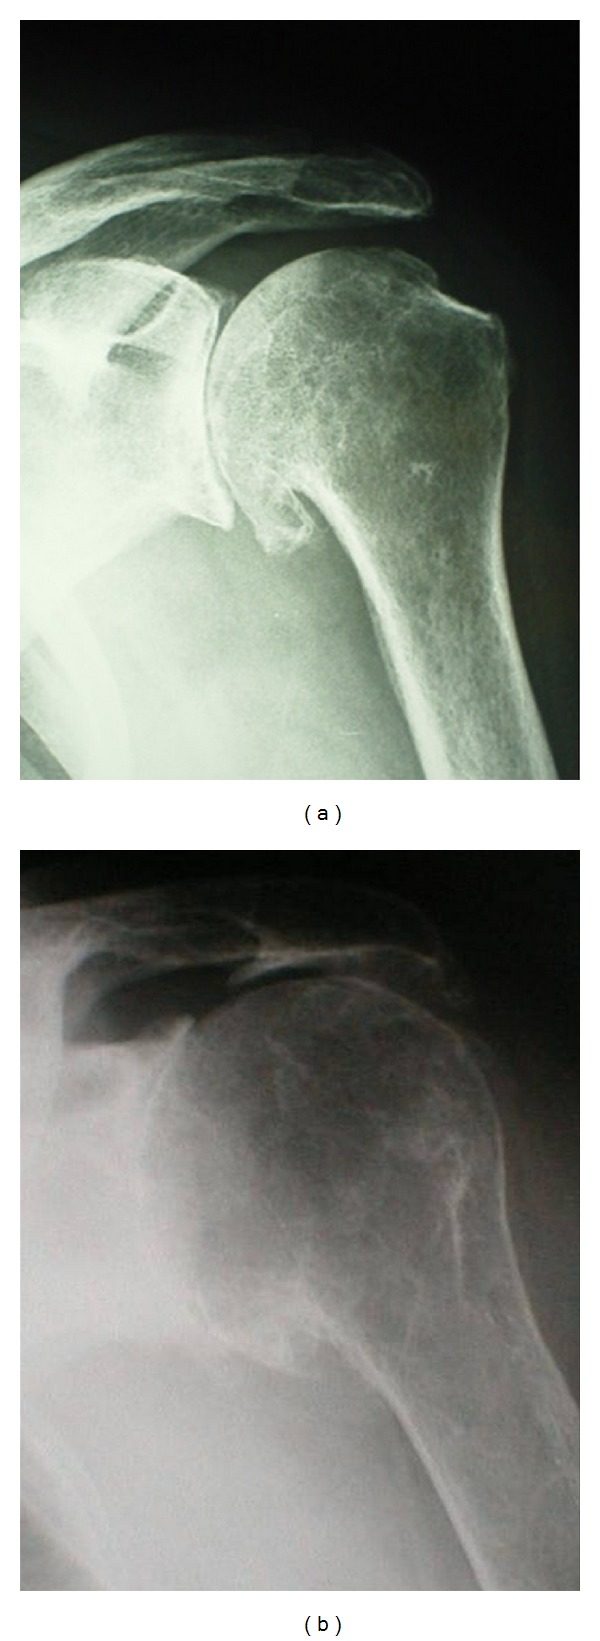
Shoulder OA: radiographic appearance with (a) and without (b) an intact rotator cuff.
